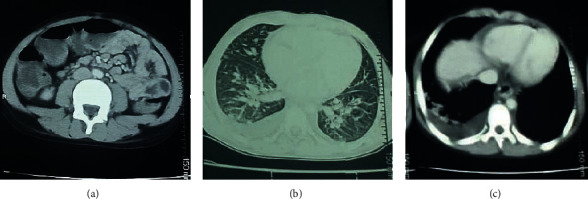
CT scan images showing (a) inflammation of the small bowel, air-fluid levels, reactional mesenteric lymphadenopathy, and (b), (c) right-sided pleurisy with bilateral pulmonary consolidation.
